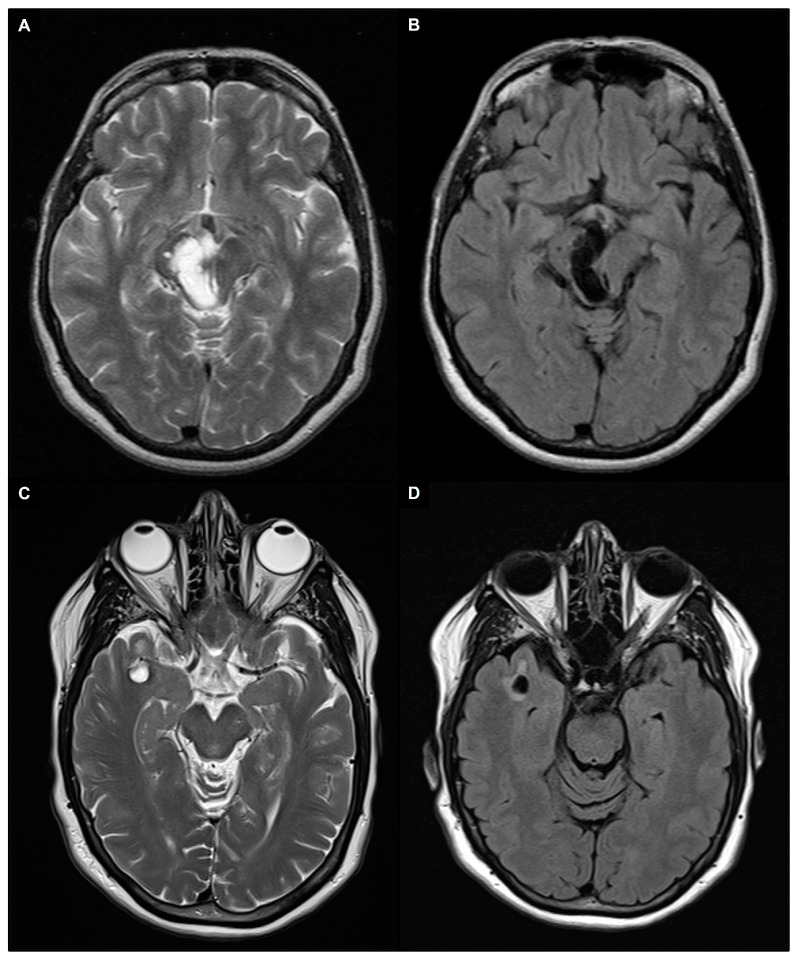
A 1.5 T magnetic resonance imaging appearance of giant PVS in T2 turbo spin echo images (A,C) and fluid attenuated inversion recovery (FLAIR) images (B,D). (A,B) Patient with giant PVS in the right mesencephalothalamic region (type III) that cause a mass effect on the aqueduct of Sylvius. (C,D) Patient with a right anterior temporal lobe enlarged perivascular space (type IV) characterized by an elevated perilesional FLAIR signal with no mass effect, which indicates gliosis.
